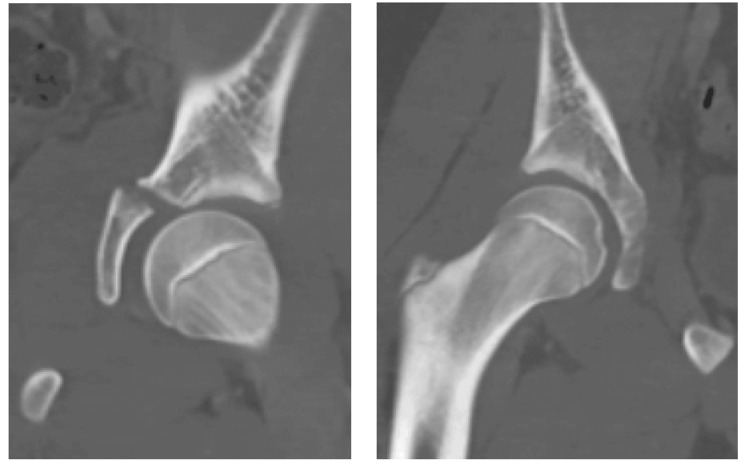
The coronal CT section of left triradiate cartilage acetabular fracture shows nonconcentric positioning of the hip with displacement appreciated.
